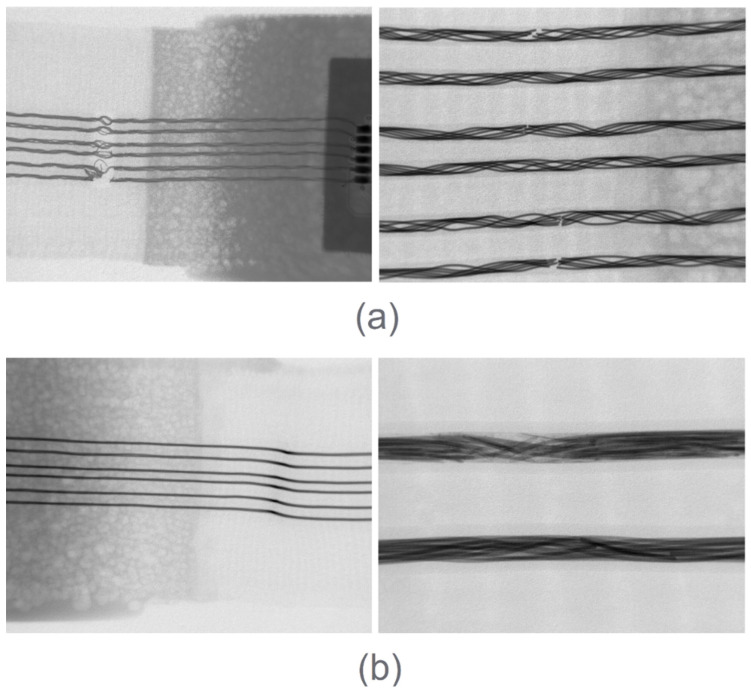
X-ray inspection photos after bending tests. (a) Yarn 1 after bending tests. (b) Yarn 2 after bending tests.
